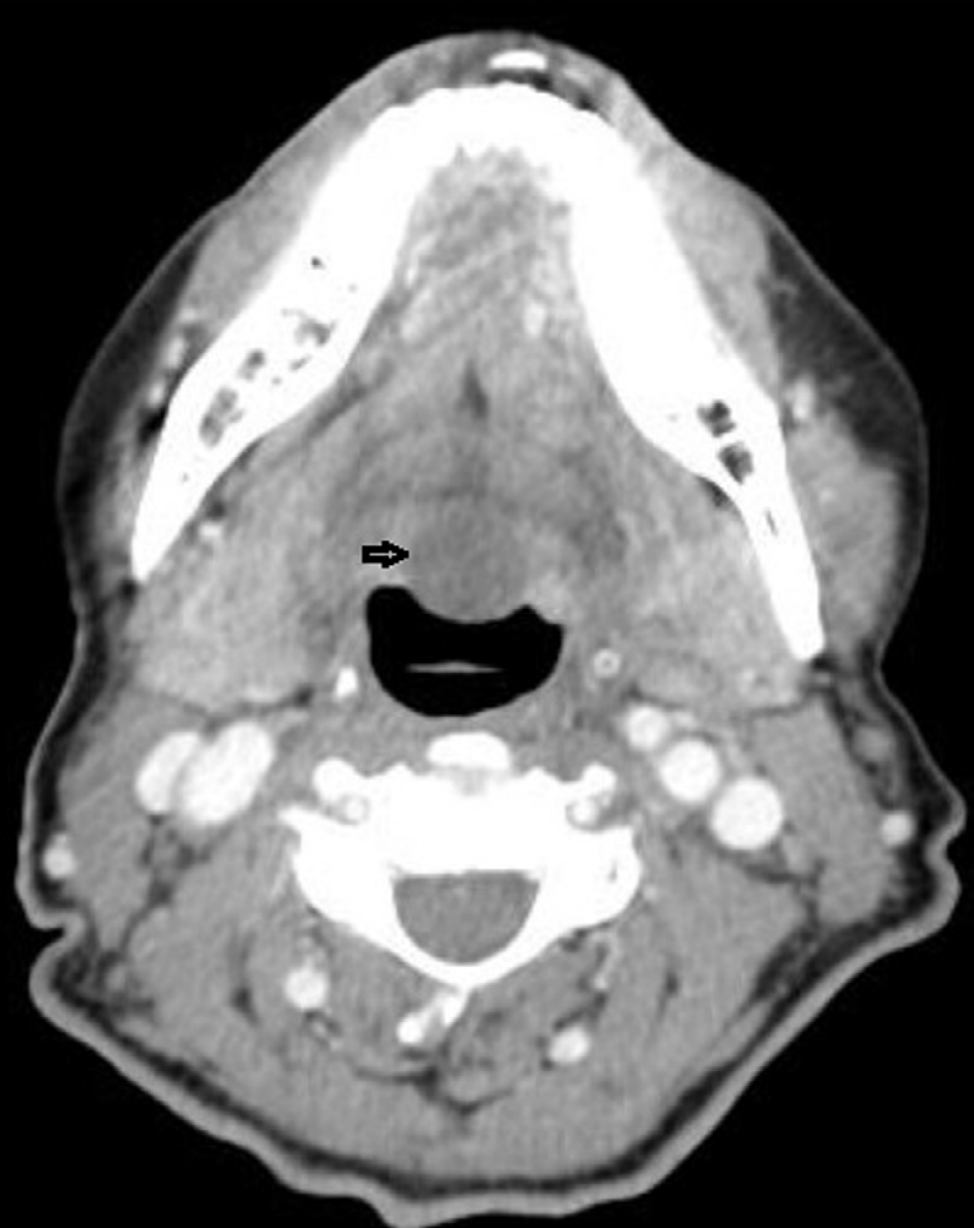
CT scan (axial plane)—homogeneous 22 × 18 mm nodular formation in the posterior region of the base of the tongue
